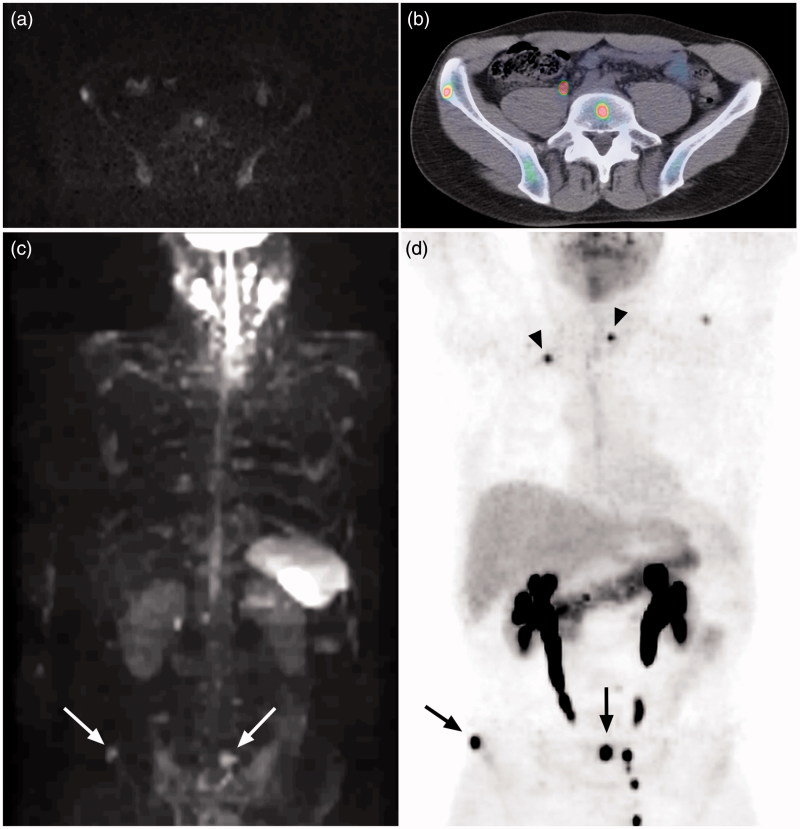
Bone marrow metastases in the lumbar spine and pelvis clearly visualized by both axial diffusion-weighted MRI (a) and 5-HTP PET/CT (b). The lesions in (a) and (b) are indicated (arrows) in MIP-images of diffusion-weighted MRI (c) and 5-HTP PET (d) in the same patient. The lymph node metastasis in thorax and bone marrow metastasis in vertebra Th II (arrow heads) are not detected with MRI (c), but clearly seen with PET (d). Hyperintense normal CNS, salivary glands, spleen, and lymph nodes are also seen in (c). Normal excretion of metabolites to the urinary tract is seen in (b) and (d).
